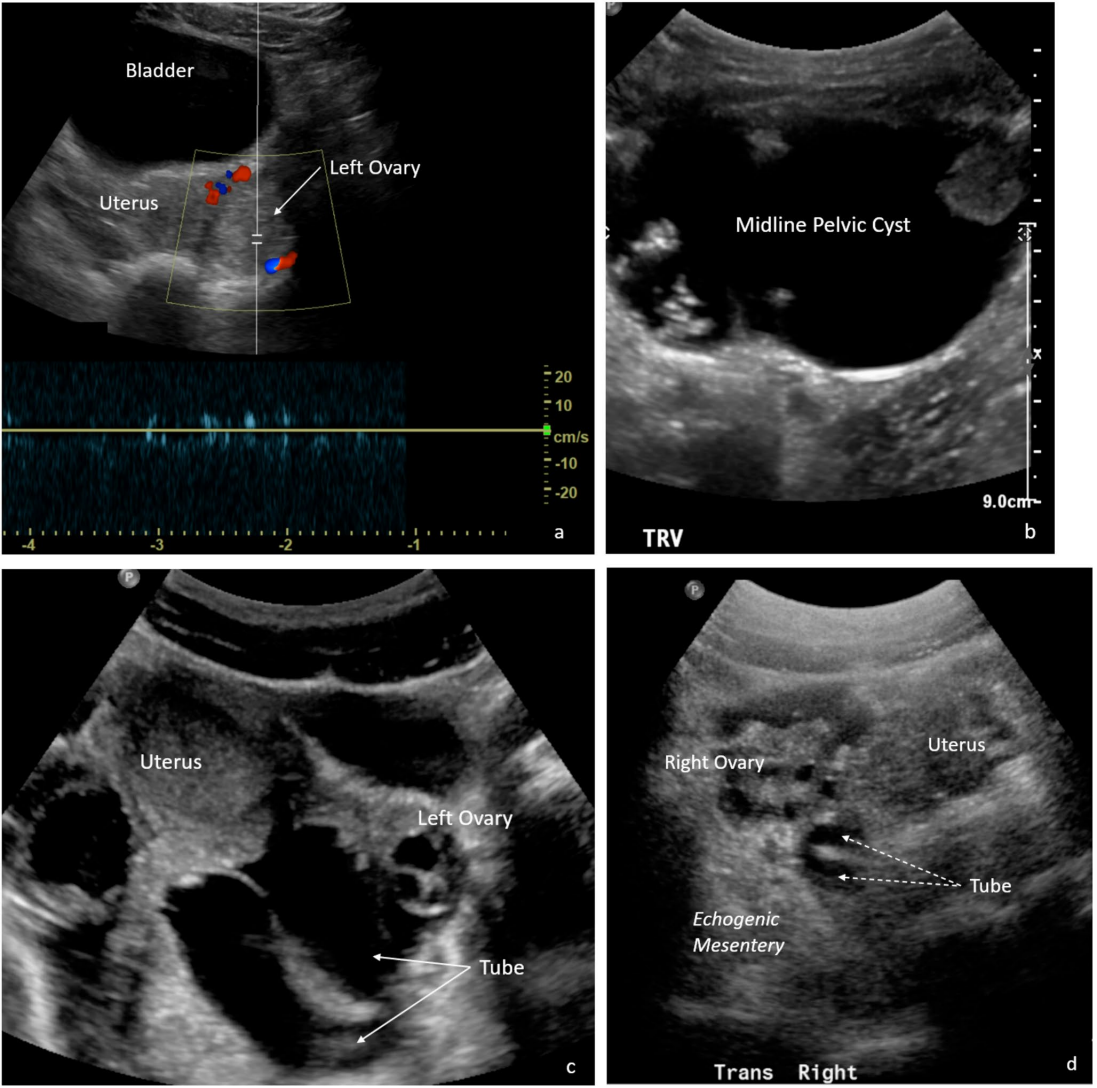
False positive ultrasound (US) in a 17-year-old girl with left pelvic pain shows a large left ovary with decreased to absent spectral Doppler signal (a); at surgery, this was a hemorrhagic cyst. Equivocal US in a 9-year-old girl with concern for abdominal mass shows an 8.4 cm complex adnexal cyst/mass in the pelvic midline, with left ovary not visualized (b); at surgery this was a left ovarian mass without torsion, which on pathology examination was a mature cystic teratoma. True positive US in a 13-year-old girl with left pelvic pain shows a fluid-filled tubular left adnexal structure (arrows) adjacent to a normal left ovary (c); at surgery, this was tubal torsion. False negative US in a 12-year-old girl with right pelvic pain was noted to have echogenic mesentery and a normal right ovary without torsion (d); at surgery, this was identified as a tubal torsion with a completely necrotic tube
